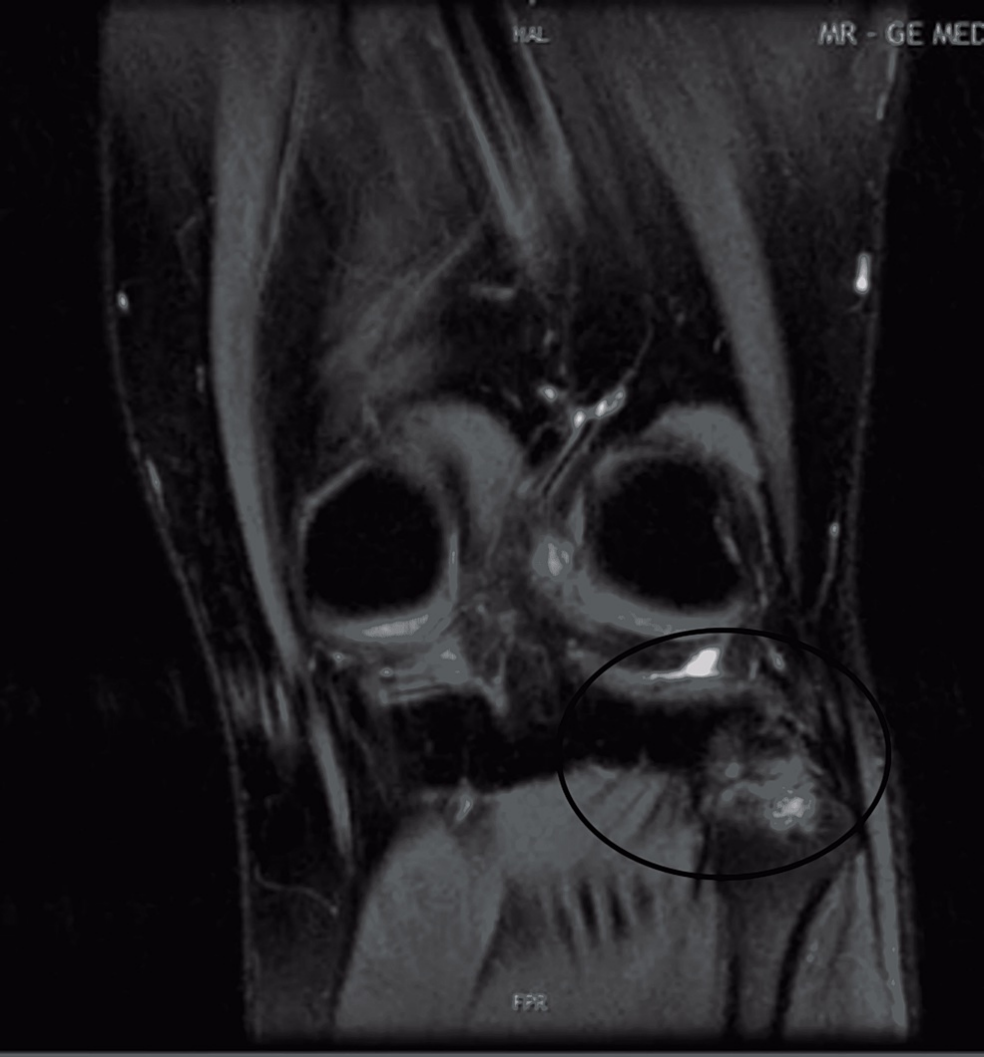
Preoperative MRI (T2 sequence) showing the osteoarthritis of the proximal tibiofibular joint
